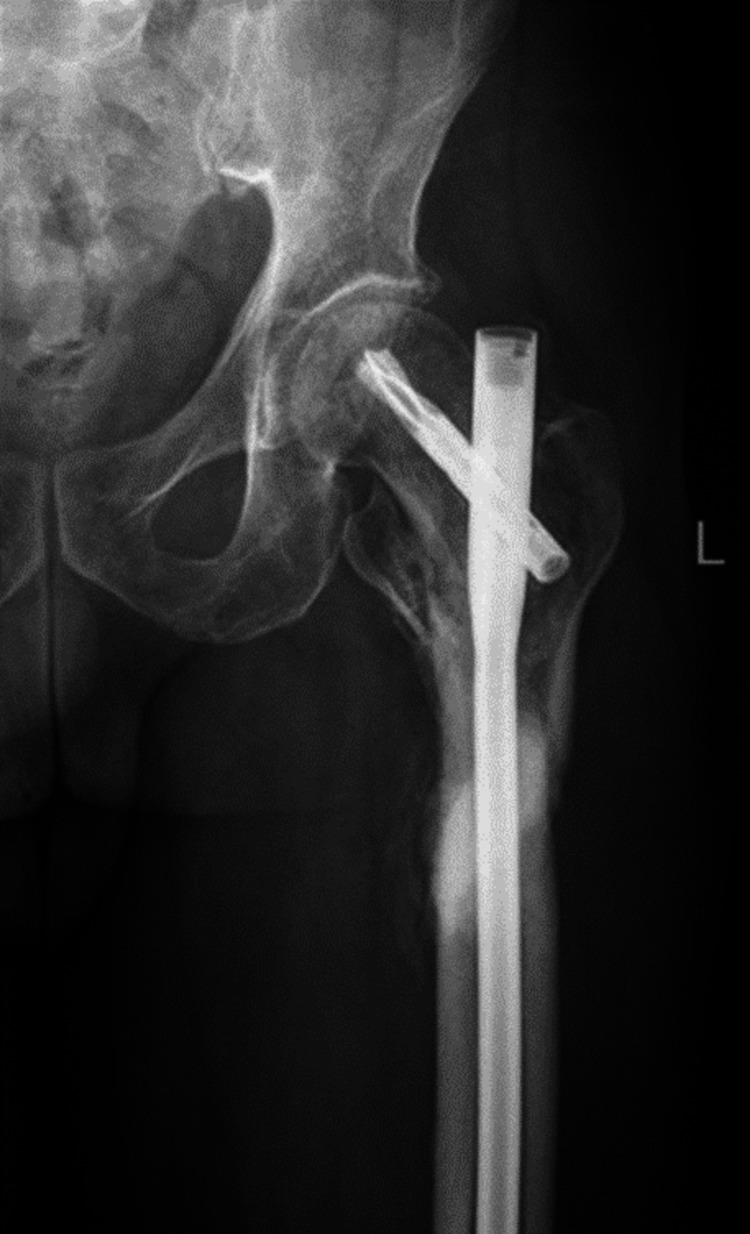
Six months follow-up radiograph
